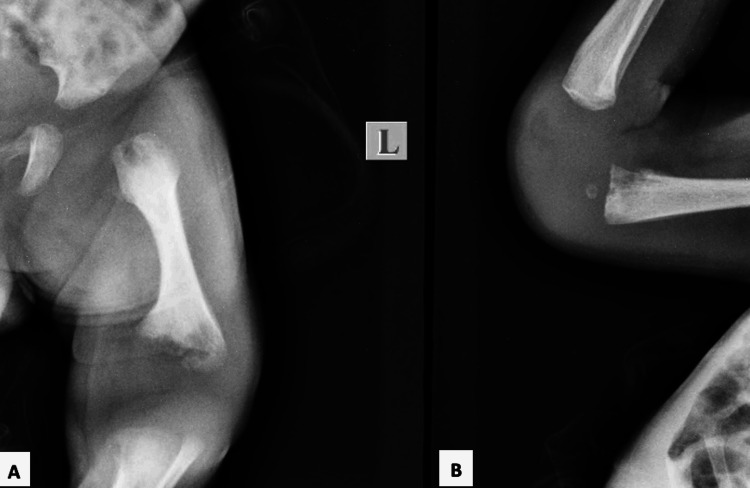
Radiograph depicting widening of joint space with the left knee joint (A) and normal elbow joint (B)
